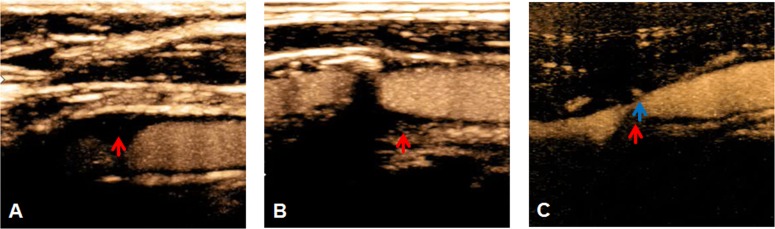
Grades of intraplaque neovascularization as assessed by contrast–enhanced ultrasound (CEUS). The arrows indicate specific plaque characteristics. (A) Grade II: very low diffuse enhancement, no focal intraplaque enhancement. (B) Grade III: clear focal plaque shoulder enhancement in proximal part of carotid plaque. (C) Grade IV: clear focal plaque shoulder enhancement (blue arrow) with strong diffuse intraplaque enhancement in an additional region (red arrow).
